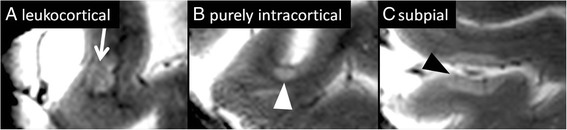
Cortical gray matter lesions in multiple sclerosis. Cortical gray matter lesions can be differentiated into distinct lesion subtypes on 7 T T2*w images. Leukocortical (type I) lesions (a) cross the border (*white arrow*) between the white and the gray matter. Purely intracortical (type II) lesions (b) are commonly small and centered on a small blood vessel (*white arrowhead*). Finally, subpial (type III/IV) lesions (c, *black arrowhead*) grow from the subpial cortical area into the cortex. *The purely intracortical (type II) lesion depicted in this figure has previously been published in: “Ultrahigh field MRI in context of neurological diseases.” Kuchling J, Sinnecker T, Bozin I, Dörr J, Madai VI, Sobesky J, Niendorf T, Paul F, Wuerfel J. Nervenarzt. 2014;85(4):445–58. doi:*
